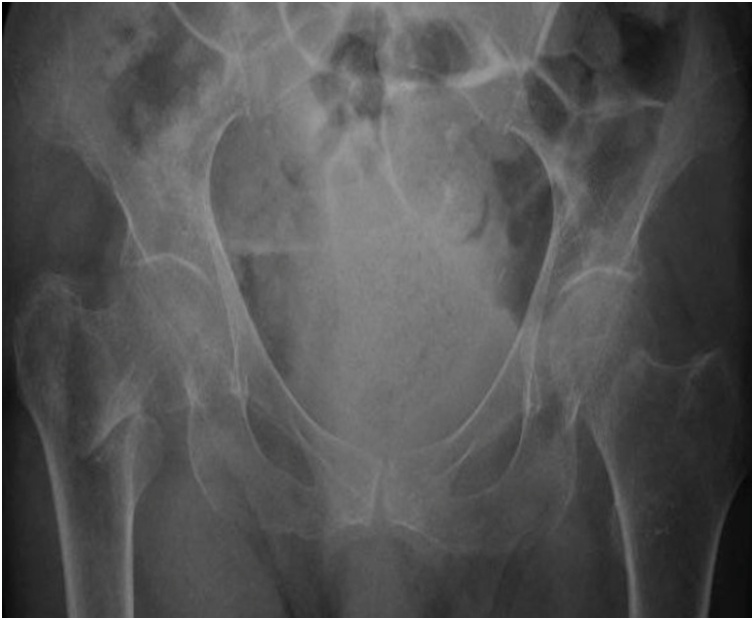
Preoperative radiographic examination of both hips in AP view of a patient with bilateral simultaneous hip fracture with different pattern showed a trochanteric fracture of the right and a subcapital fracture of the left.
